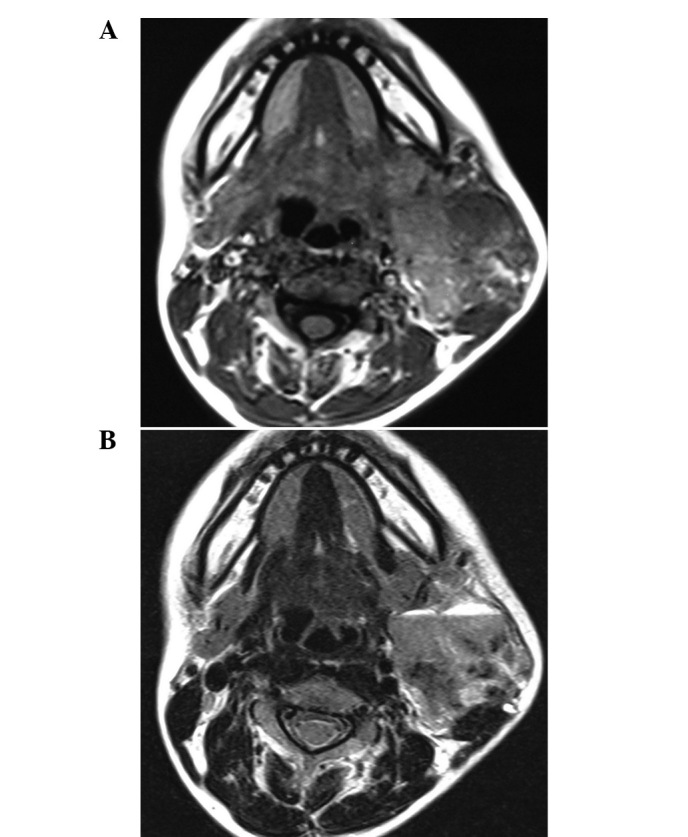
Type II BCC with repeated infection. (A) A heterogeneously isointense and hyperintense mass with an irregular border presented in the left side on T1WI. (B) The cystic section of the mass was narrowed and markedly hyperintense on T2WI. BCC, branchial cleft cyst; T1WI, T1-weighted image; T2WI, T2-weighted image.
